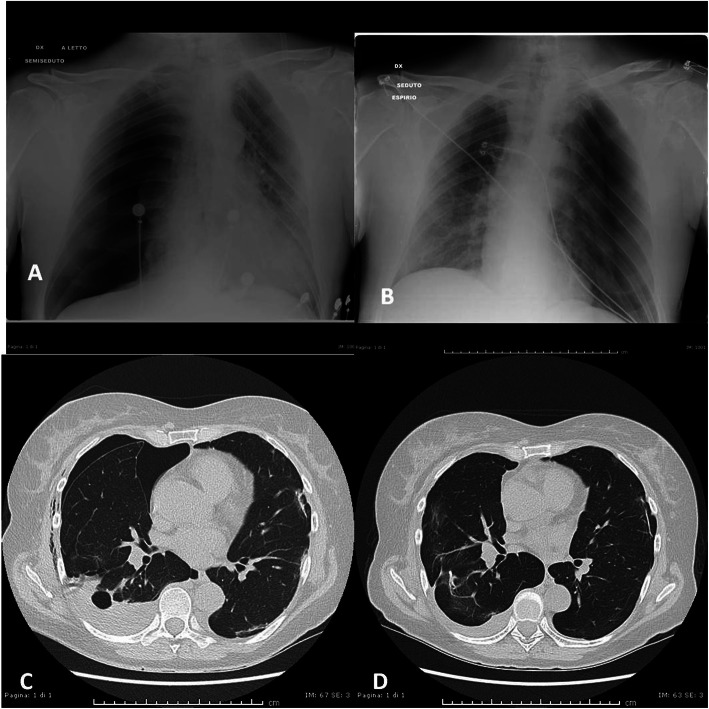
Chest X-ray showing massive left (a) and right (b) pneumothorax in COVID-19 patient 1; preoperative CT scan showing right pneumothorax with bulla of the apical segment of the lower lobe (c) and ground glass opacities (d) typical of COVID-19 disease in patient 2
